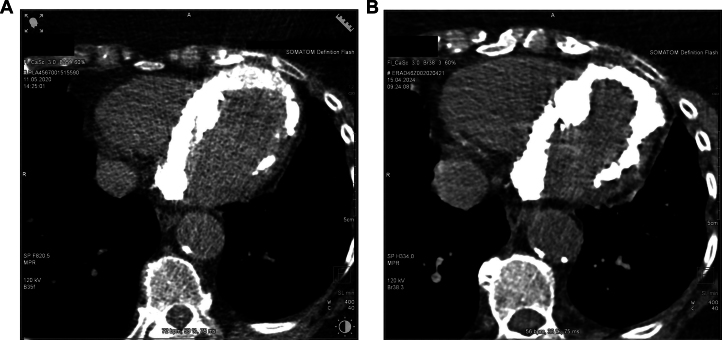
Cardiac Computed Tomography of Myocardial Calcification Cardiac computed tomography showing massive myocardial calcification of the left ventricle (A) and the progression within 4 years (B).
